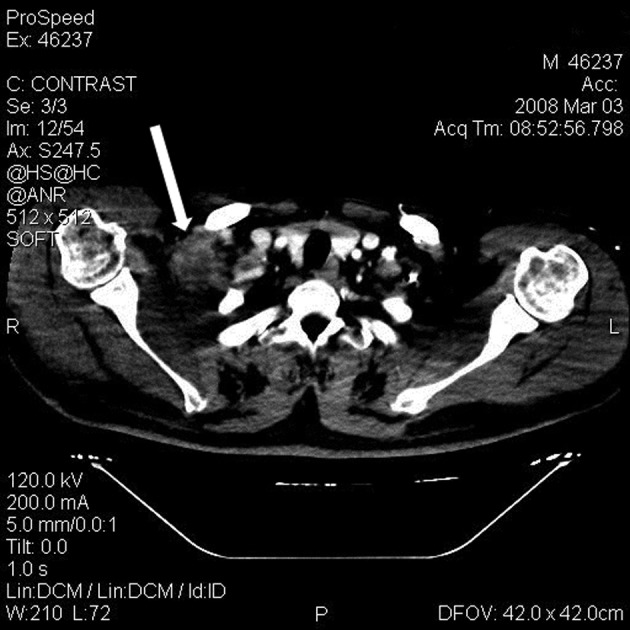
Post-ablation shrinkage of the mass (arrow) by half its size (3 cm) at the one-month follow-up computed tomography (CT) scan with the patient in supine position.
